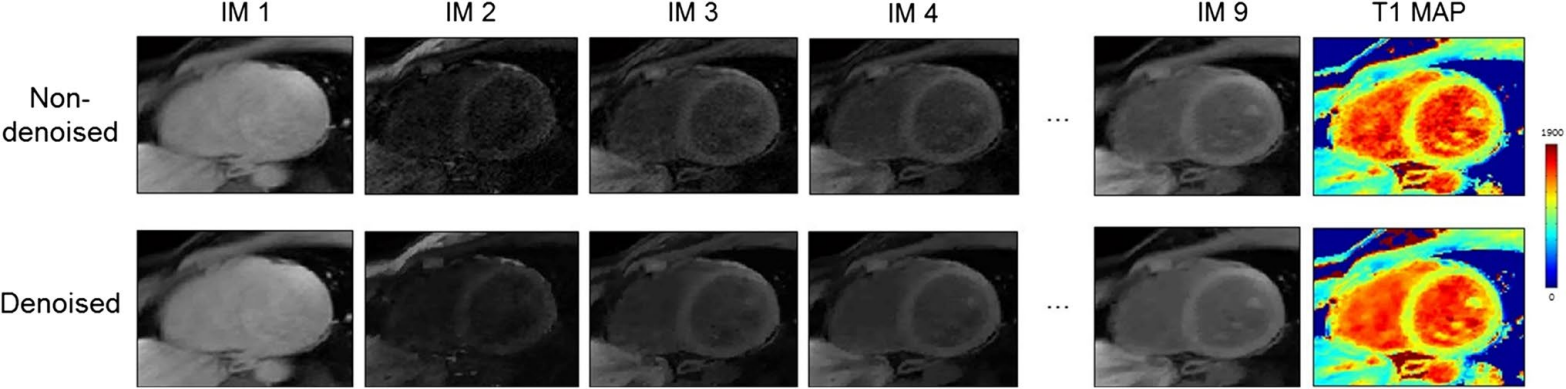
Single T1-weighted images before and after 3D Beltrami denoising and the corresponding 3D SASHA T1 map for a representative subject
